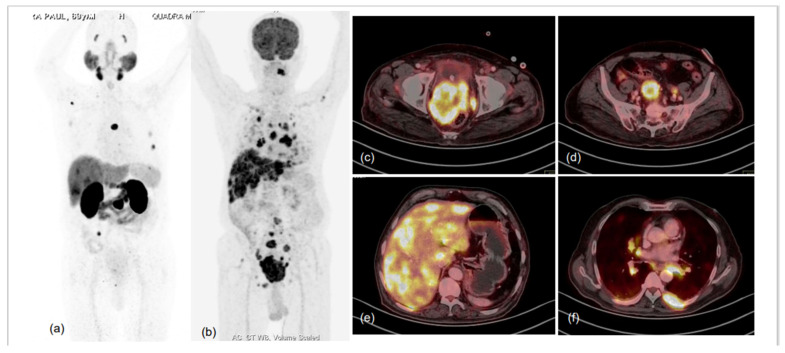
76-year-old male, attended urologist’s office for lower urinary tract symptoms (LUTS) and prostatomegaly; suspected prostate carcinoma, underwent biochemical evaluation, and serum PSA was 1.120 ng/mL. TRUS-guided prostate biopsy was performed and was diagnosed as high-grade acinar adenocarcinoma (Gleason score 5 + 5 = 10); IHC tumour cells were found to express cytokeratin, EMA, TTF1, synaptophysin, chromogranin A and Mib-1-labelling index of approx. 75%. Hence, a diagnosis of high-grade acinar adenocarcinoma of prostate with small-cell transformation was made and the patient was taken for dual-tracer PET with ^68^Ga-PSMA-11 and FDG after deliberate discussion with referring urologist and taking him and the patient into confidence. MIP images of ^68^Ga-PSMA-11 (a) and FDG (b) PET CT scans demonstrated significant discordance in terms of number and intensity of tracer uptake in the lesions, with significantly more lesions showing FDG uptake of increased intensity. Fused trans-axial PET and CT slices of FDG PET-CT (c–f) showed FDG-avid lesions with high-grade metabolic activity with visceral (hepatic) and lytic skeletal lesions. The results were in accordance with the known phenomenon of small-cell prostate carcinomas being more aggressive, showing lesser PSMA expression in lesions and being metabolically active due to rapid proliferation.
